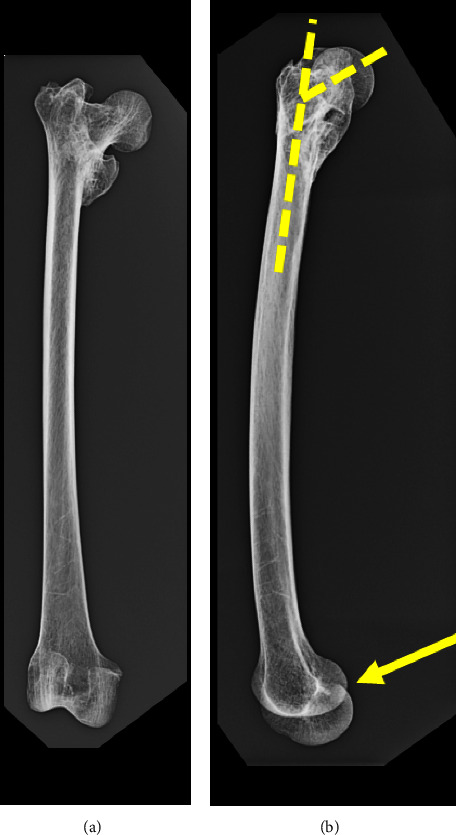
Radiographs of the entire femur (This is an original figure created by the authors of the manuscript). (a) Anteroposterior projection. (b) Lateral projection. Note that with the femoral condyles rotationally the same (solid yellow arrow), there is retroversion of the femoral head/neck relative to the shaft (hatched lines).
